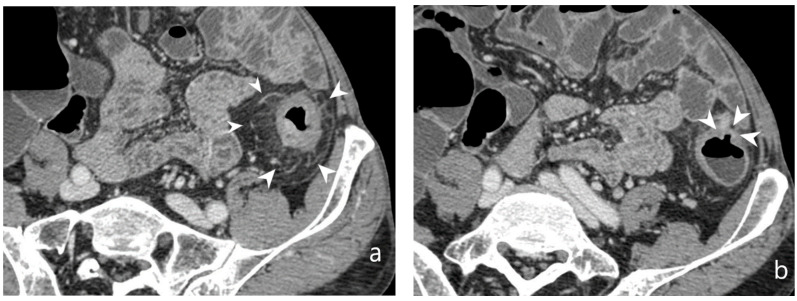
Assessment of disease activity. Axial CT-E after iodinated contrast injection and administration of polyethylene glycol solution (a,b) shows thickening of the descending colon with perivisceral fat stranding surrounding the pathological segment (white arrowheads in (a)). A bowel wall ulcer is also evident in another plane (white arrowheads in (b)). Original figures by LMM and LL.
